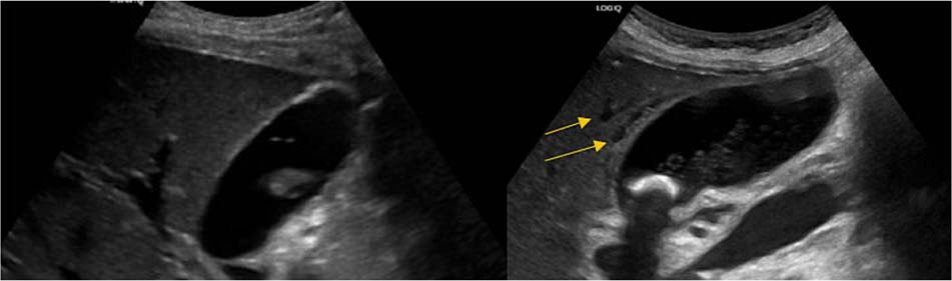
Pericholecystic fluid (arrows) and positive sonographic murphy sign present on the post-HIDA ultrasound (right) that was not previously seen on the pre-HIDA ultrasound on admission (left).
